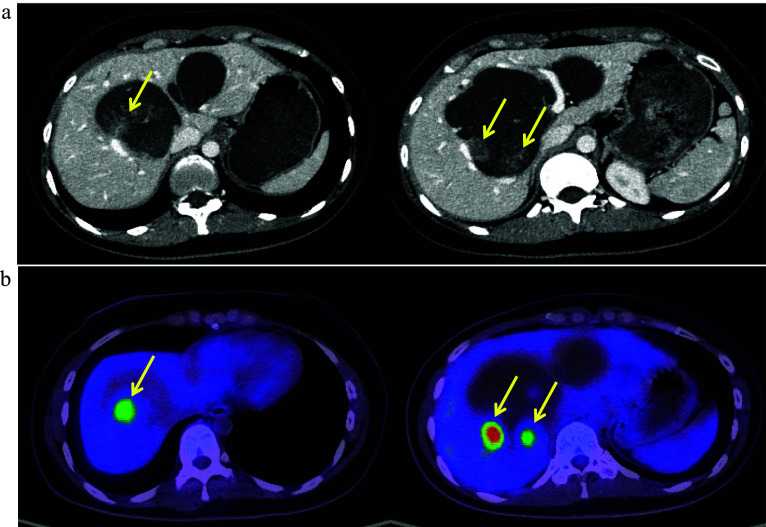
CT scan and FDG-PET. CT scan revealed three papillary tumors in the right hepatic duct (17, 21, and 11 mm from the left), and these lesions showed increased ^18^F-FDG accumulation (SUV_max_ = 14.1) on positron emission tomography (arrows). Neither lymphadenopathy nor distant metastasis was detected (a CT scan, b FDG-PET)
